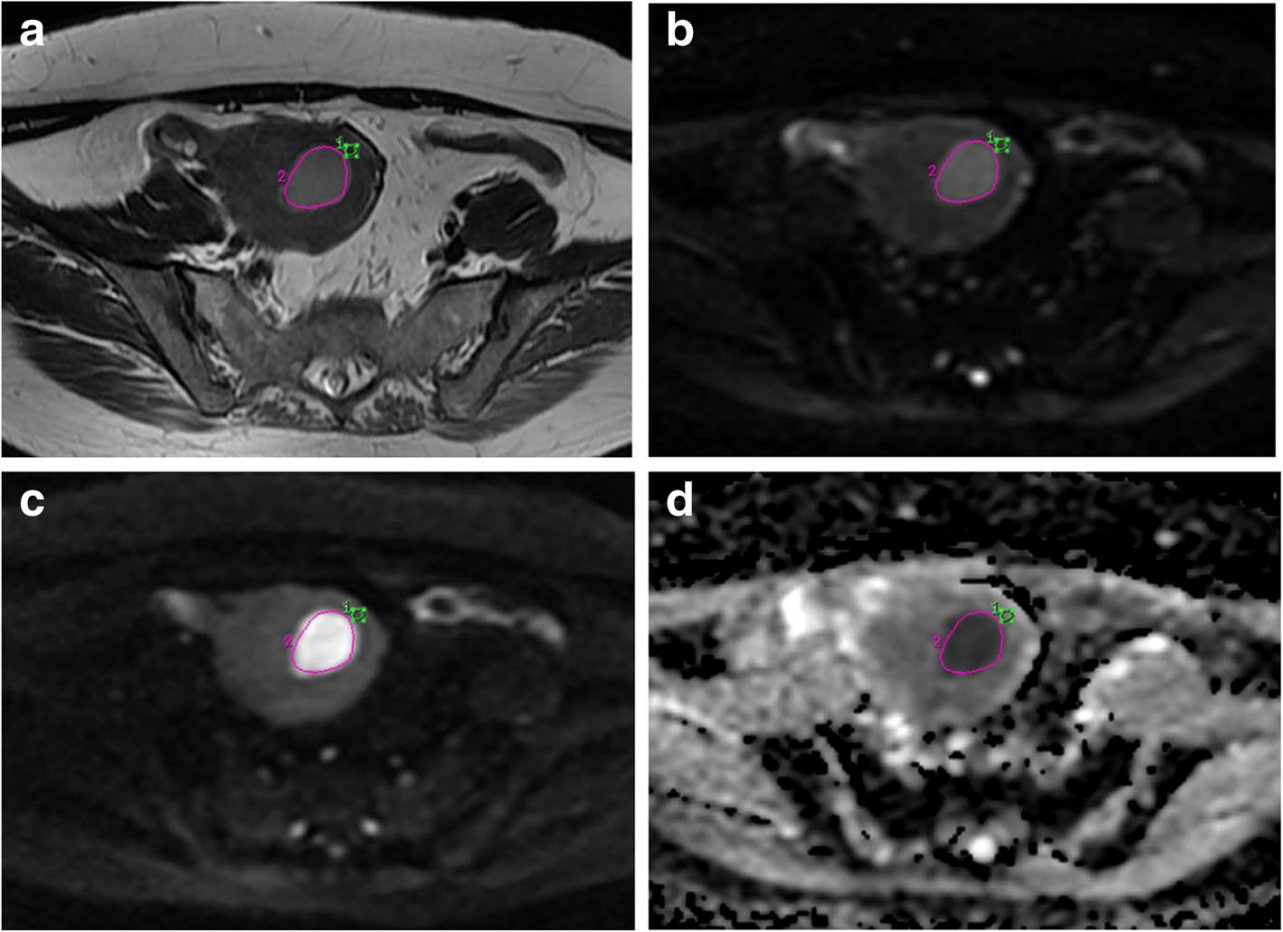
Endometrial adenocarcinoma with superficial myometrial invasion in a 43-year-old woman. An elliptical region of interest (ROI) was placed on peritumoral zone (ROI_1_ with green color), which was assessed subjectively on a cross-section of the T2-weighted image obtained by echo planar imaging (*b* = 0 s/mm^2^). In addition, a freehand ROI (ROI_2_ with pink color) was placed on the tumor which contained the largest endometrial cancerous area, avoiding artifacts from the neoplastic/non- neoplastic interface and visible lesions or vascular structures in the myometrium. a T2-weighted image; b diffusion-weighted magnetic resonance image (*b* = 0 s/ mm^2^); c diffusion-weighted magnetic resonance image (*b* = 1000 s/mm^2^); d apparent diffusion coefficient map
